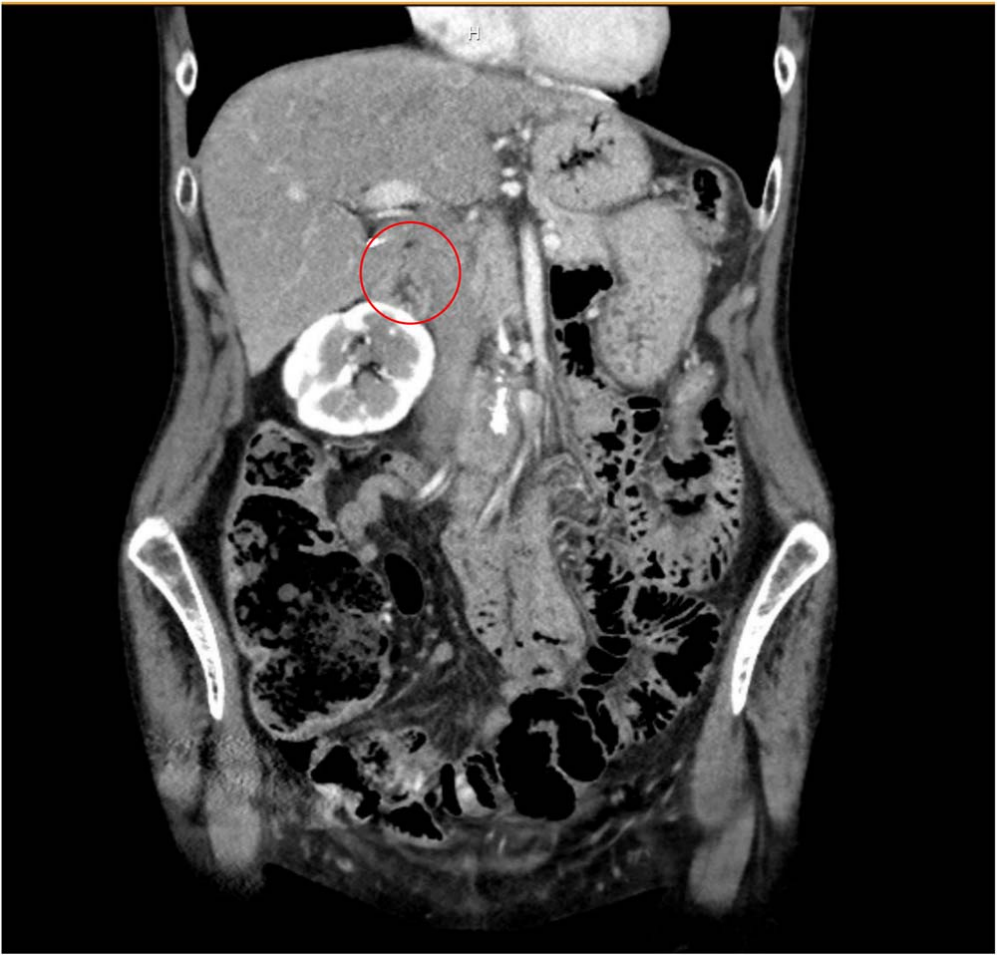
Coronal CT of abdomen and pelvis noting a 1.3 cm right adrenal mass (circle).
